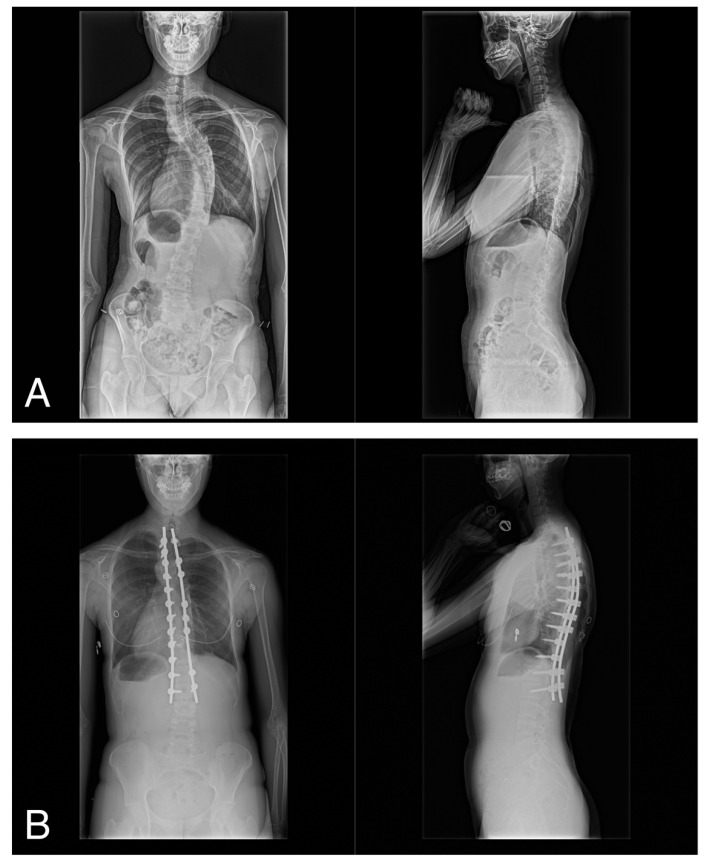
(A) Preoperative anteroposterior and lateral views of a 17-year-old woman with Lenke type 1A scoliosis, and (B) anteroposterior and lateral radiographs at 2 years postoperatively.
